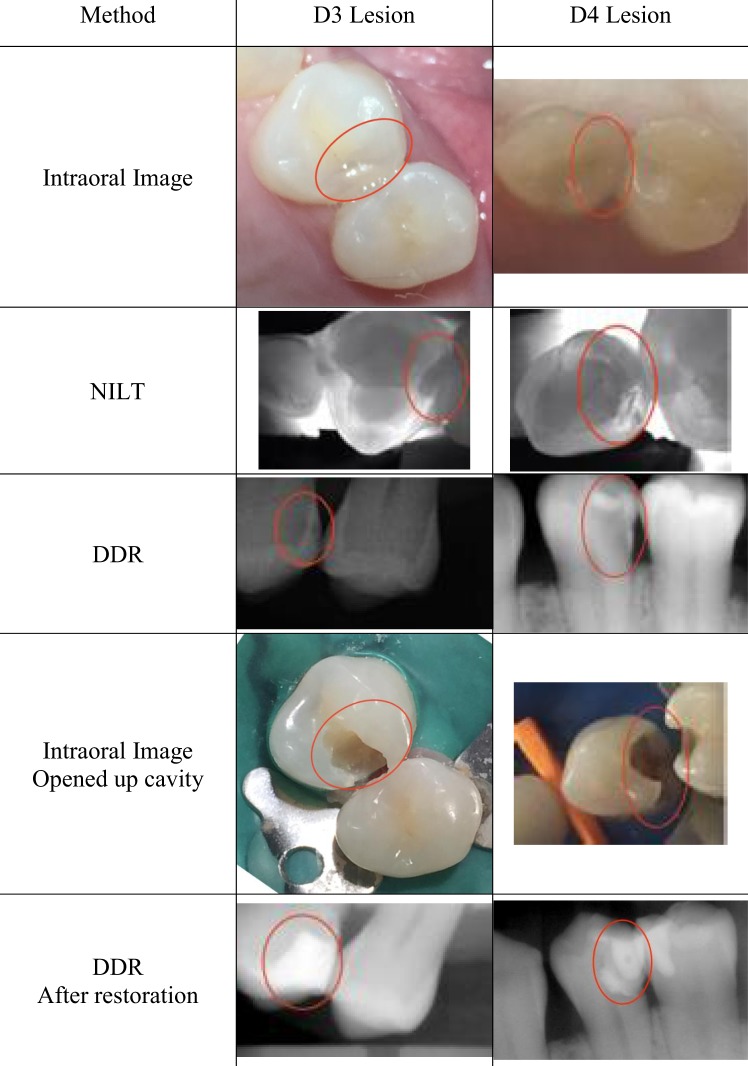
Examples of D3 and D4 lesions for each method: Intraoral image, NILT: Near infrared light transillumination, and DDR: Direct digital radiography. Orange circles indicate the location of lesion. D3 lesion was confirmed on the distal surface of the maxillary left first premolar. D4 lesion was confirmed on the distal surface of the mandibular left first premolar.
